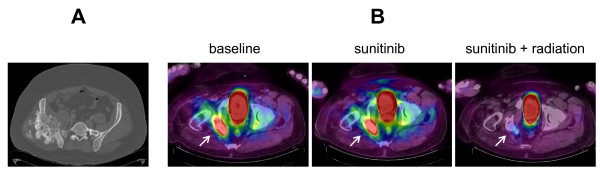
Representative scans done upon diagnosis and during the course of sunitinib treatment. (A) Diagnostic computed tomography showing the multilobulated chondrosarcoma mass arising from his right pelvis. (B) Superimposed fluorine-18- fluorodeoxyglucose-positron emission tomography and computed tomography scans showing the pelvis mass (white arrow) immediately prior to sunitinib, two months after initiation of sunitinib alone and two months after combined sunitinib and proton beam radiation. Intensity of fluorine-18-fluorodeoxyglucose uptake: red, high; yellow, intermediate; blue, low.
